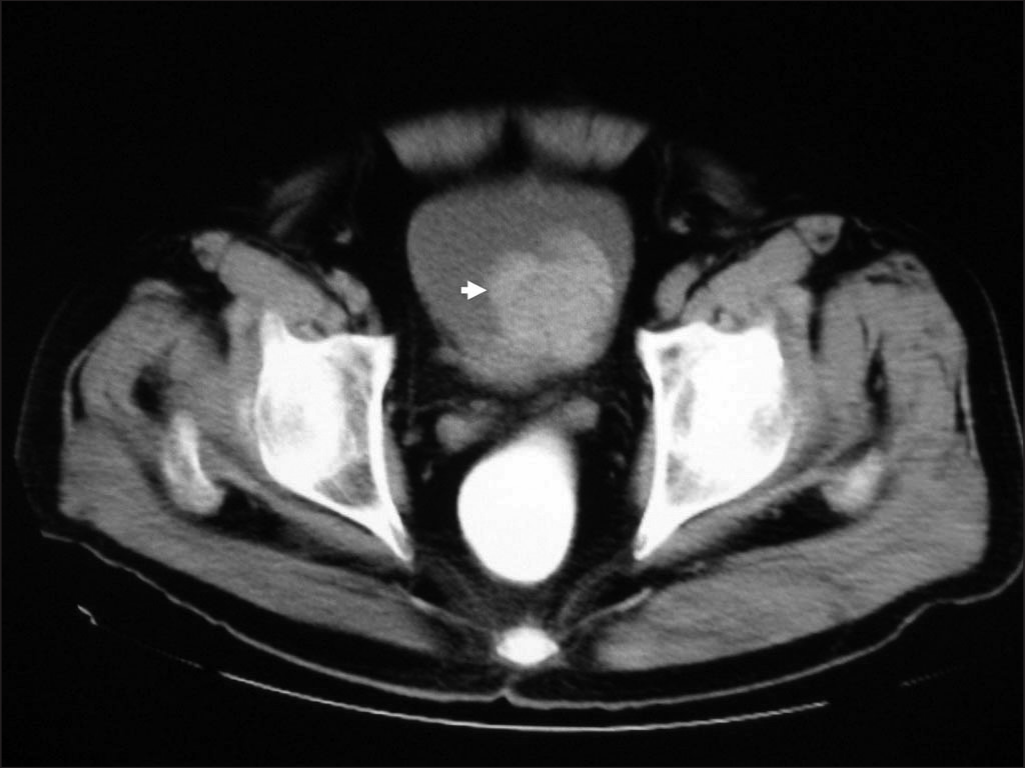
Contrast-enhanced CT scan showing moderately enhancing lobular intravesical mass lesion (arrow) arising from the base of the urinary bladder, involving the right vesicoureteric junction.
